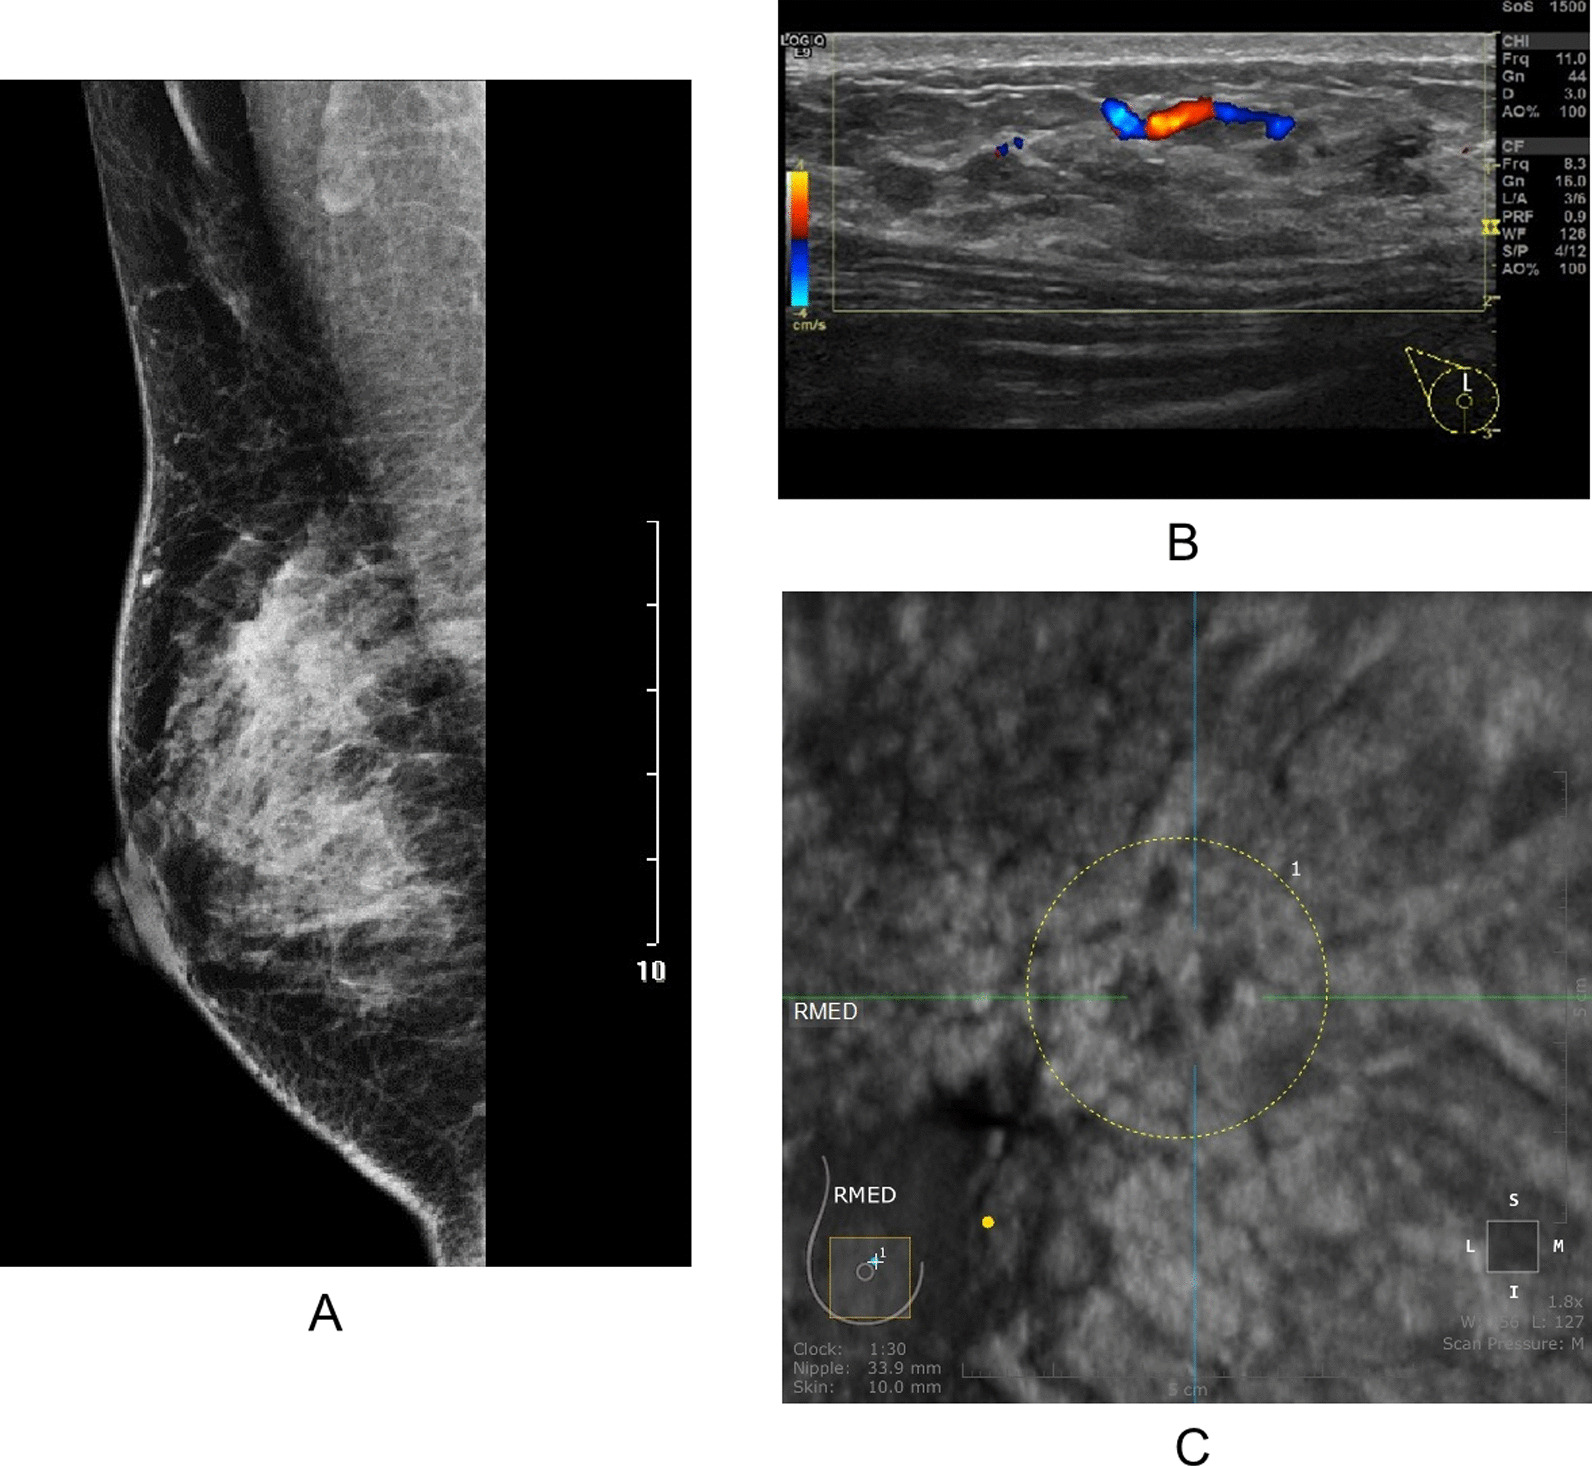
Hand-held ultrasonography and automated breast ultrasonography non-mass-like lesion findings with asymmetry (MG). A Large irregular block shadow with fuzzy and rough boundary and a large number of burrs like shadows of different lengths could be seen in the upper right breast area of MG, ACR BI-RADS 4A. B HHUS showed a patchy heterogeneous echo area in the right breast with irregular thickened duct structure. The thickened duct was hypoechoic. CDFI showed a little color blood flow signal in this area, Adler Grade 2, IA grade (K.O), ACR BI-RADS 4B. C The coronal plane of ABUS in the right breast showed patchy and uneven echo area with irregular shape, fuzzy edge and uneven internal echo. ACR BI-RADS 4B. Pathology:Intraductal carcinoma
